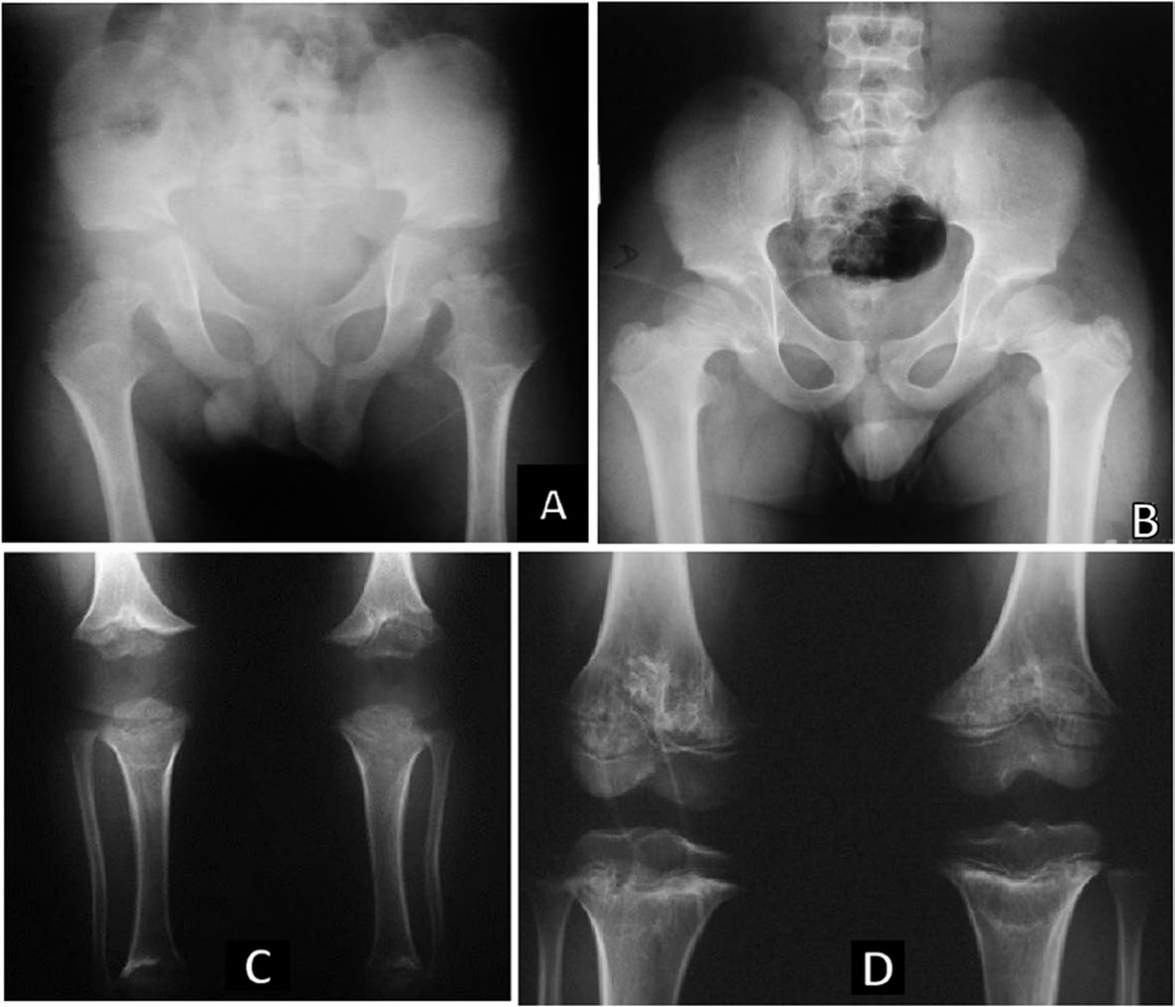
Radiographic images of children with achondroplasia at the ages of 4 (A and C) and 8 years (B and D). A-B Note squared configuration of the pelvis that remains with the years, as well as the narrowness of the sciatic notch. The femoral neck became short. In the lower long bones, the irregularity of metaphysis of the knees shows, with the age, the “chevron” shape of the distal region of femora. C-D The tibia is broad and the fibulae greater than the tibia
